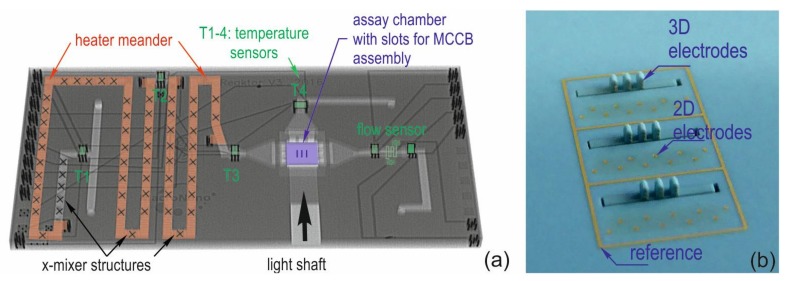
(a) Ceramic bio-reactor. The colored X-ray image makes it possible to view the integrated mixer, temperature control and flow monitoring. The base plate consists of glass and is mounted with epoxy at the bottom, next device generation with integrated 3D sensors is equipped with a ceramic base plate and slots for ceramic contact board assembly, indicated by the blue lines; (b) three miniaturized ceramic circuit boards, assembled perpendicular through slots of the ceramic base plate (LTCC test chip).
